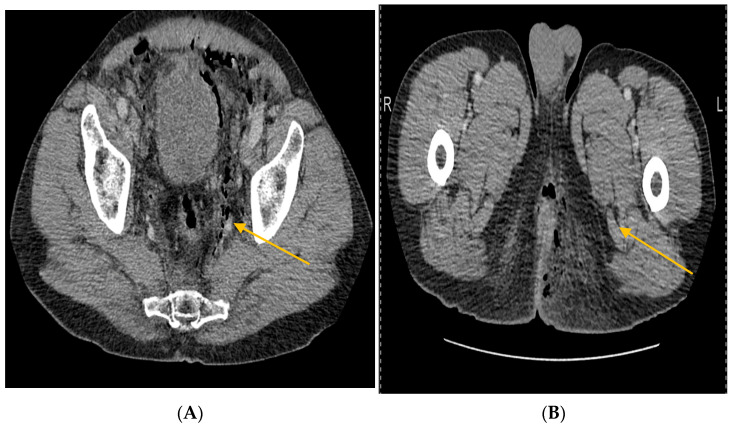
Axial CT images show bilateral ischiorectal phlegmonous collections with preperitoneal and retroperitoneal extension and free air (A-yellow arrow), and perineal soft tissue swelling with air pockets in both ischiorectal fossae, suggesting anaerobic infection with extensive necrosis (B-yellow arrow).
